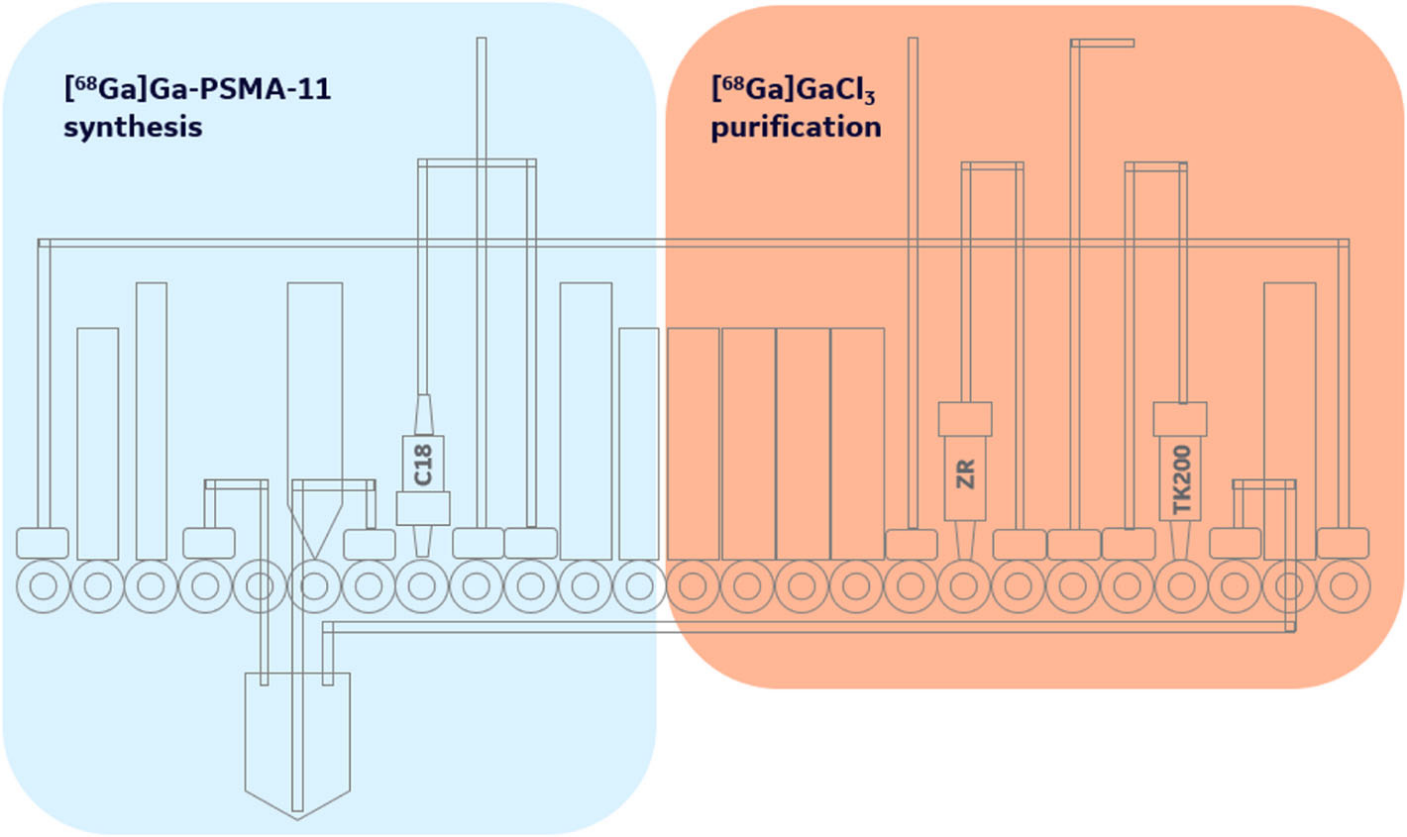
Partitioning of FASTlab cassette: Right-hand side is reserved for [^68^Ga]GaCl_3_ purification, and the left-hand side accommodates the [^68^Ga]Ga-PSMA-11 synthesis and C18 cartridge based purification
